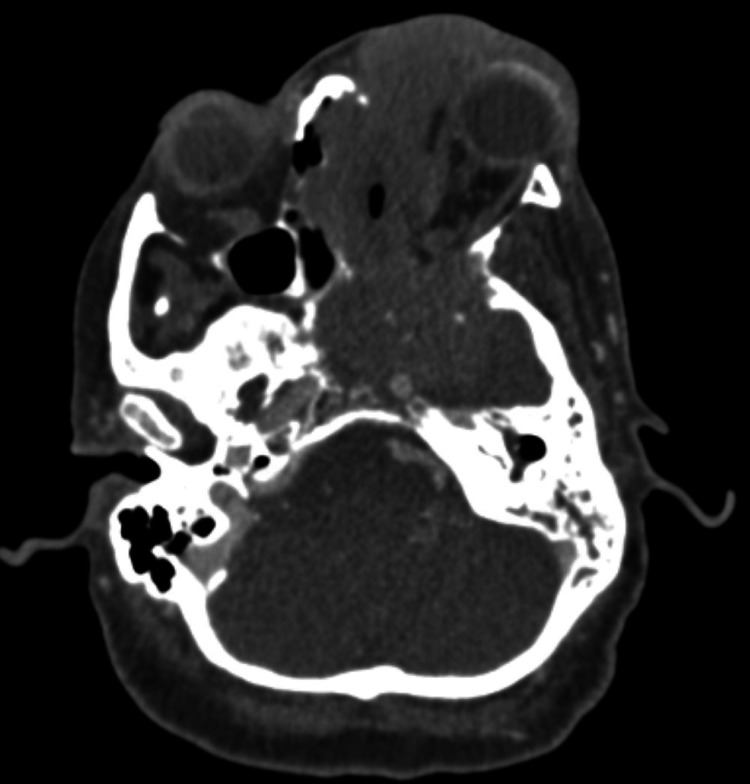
CT head with contrast A large diffusely infiltrating mass of orbital origin with prominent infiltration to the left maxillary sinus, left masticator space, left sphenoid bone, extending into the nasopharynx and invading the left nasopharyngeal soft tissues, nasal cavity, bilateral ethmoid air cells, and bilateral sphenoid sinuses. The mass extends anteriorly in the left nasal cavity and through the left face into the medial canthus region with an ulcerated aspect along the left periorbital soft tissues. There is an associated displacement of the left globe with the proptotic appearance of the left globe from mass effect with the encroachment of the mass near the fat plane along the left medial rectus.
